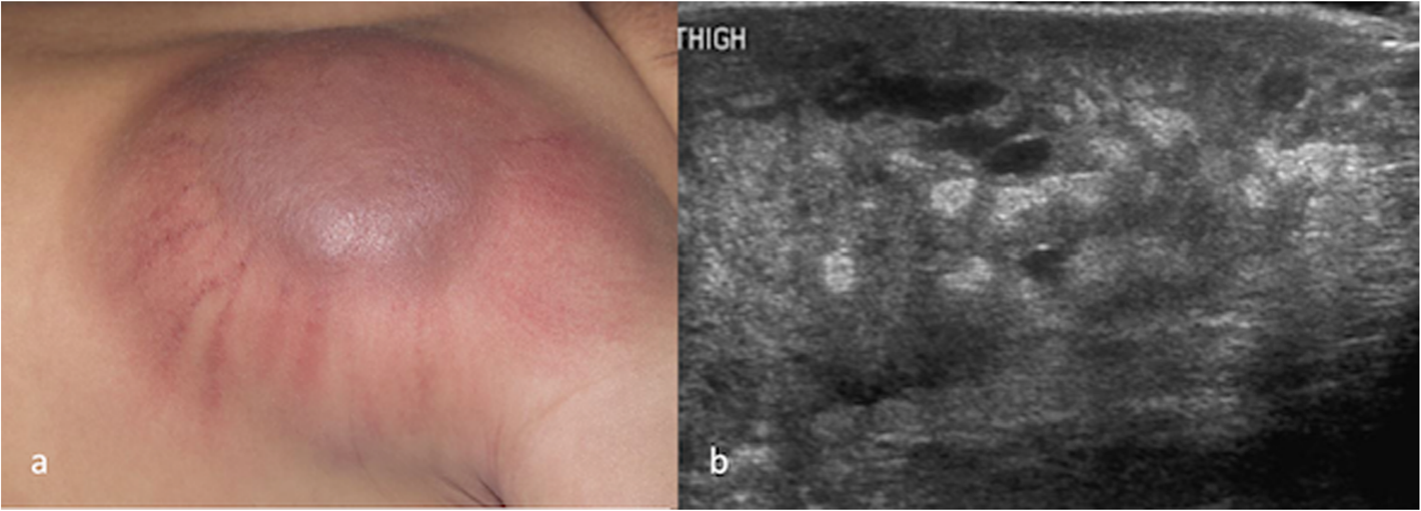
a a kaposiform haemangioendothelioma (KHE) in a 1 month old female. Clinically, the lesion appears more aggressive in nature than the common infantile haemangiomas; b an ultrasound image of a KHE in another patient, showing a far more heterogenous parenchymal echotexture than that seen in haemangiomas
